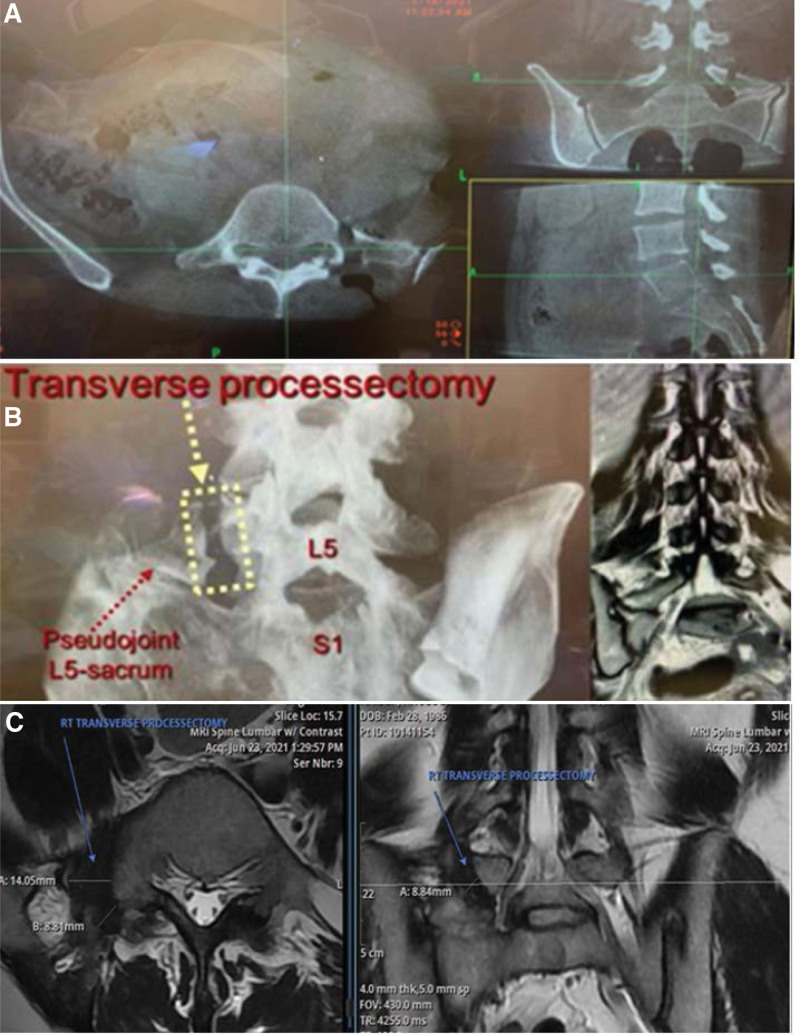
(A) Intraoperative 3-D image acquired by the *O-arm* (Medtronic^®^, USA) to confirm the complete disconnection of the enlarged transverse process (TP) from the pseudojoint sacrum region; (B) 3-D Image of the lumbar region showing the transverse processectomy (left) and preoperative lumbosacral MRI (right); (C) Postoperative lumbosacral MRI showing the transverse processectomy, axial (left) and coronal T2 image (right). TP = transverse process.
